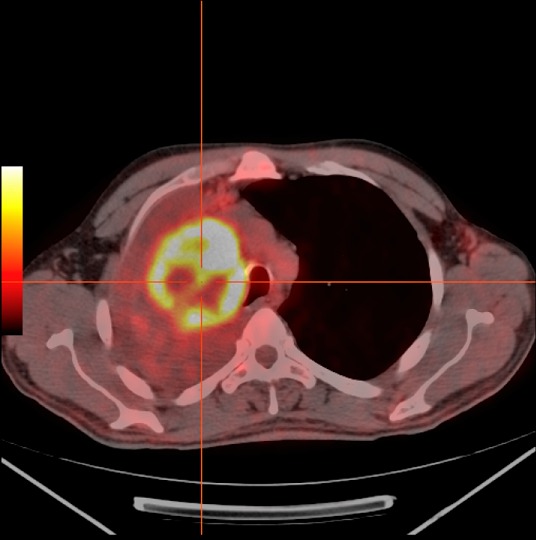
Hypermétabolisme (SUVmax bw: 9.9) sur le champ pulmonaire droit
